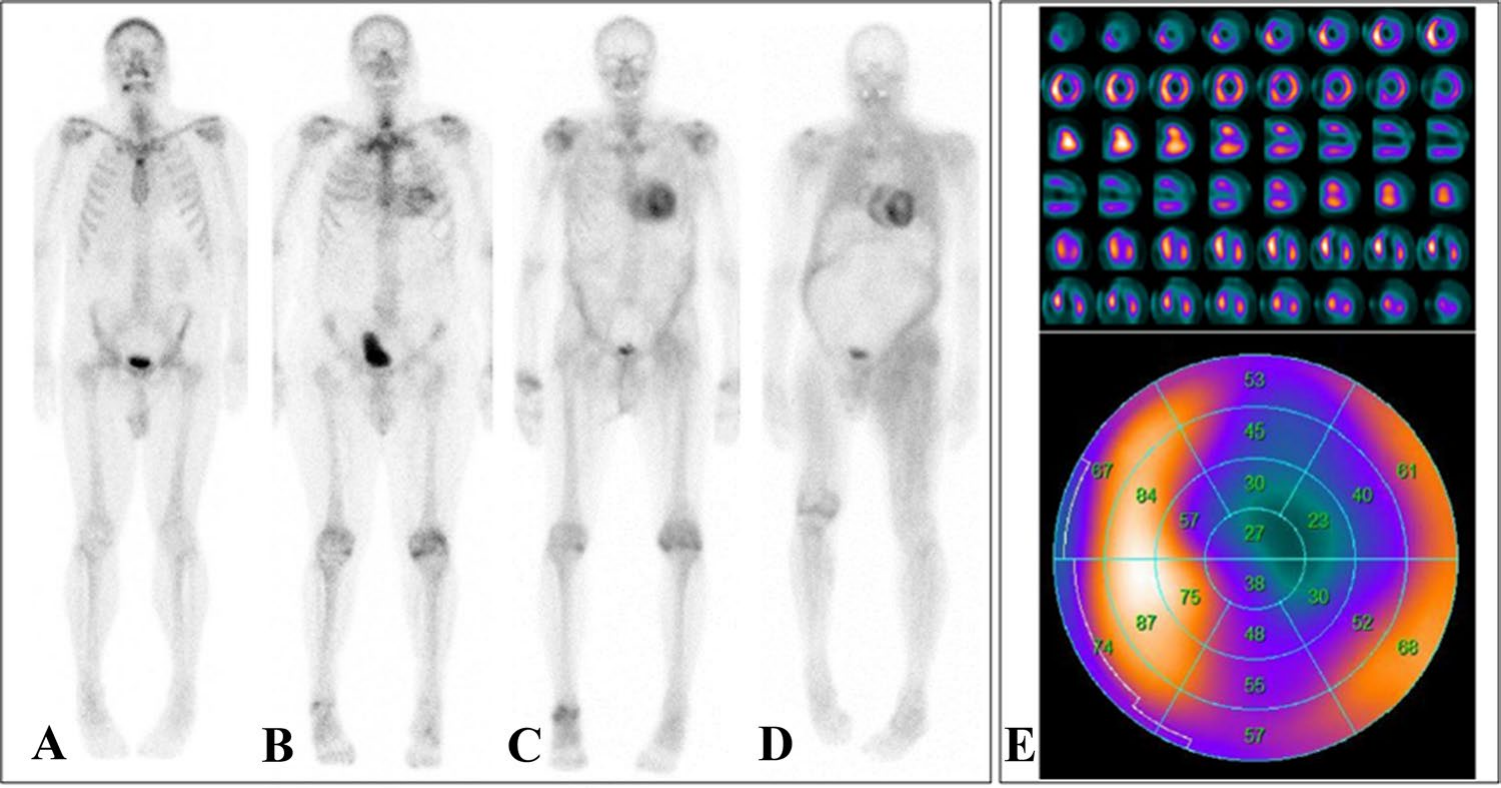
Planar 99mTc-DPD scintigraphies of four different patients with varying degrees of cardiac radionuclide uptake: No cardiac uptake (Perugini score 0, a). Light cardiac uptake with preserved delineation of bone tissue (Perugini score 1, b). Strong cardiac uptake above that of bone tissue and increased soft-tissue uptake, particularly in the shoulder, abdominal wall, and gluteal region (Perugini score 2, c). Strong cardiac and soft-tissue uptake with no discernable bone-tissue uptake, suggesting diffuse amyloid soft-tissue infiltration (Perugini score 3, d). In case of myocardial uptake on planar scintigraphy, SPECT imaging should be performed, which allows for a detailed assessment of radionuclide distribution within the left-ventricular myocardium (e, short axis, vertical long axis, and horizontal long axis slices at the top and the corresponding polar plot at the bottom; white/yellow indicates high uptake; blue/black indicates little or no uptake)
